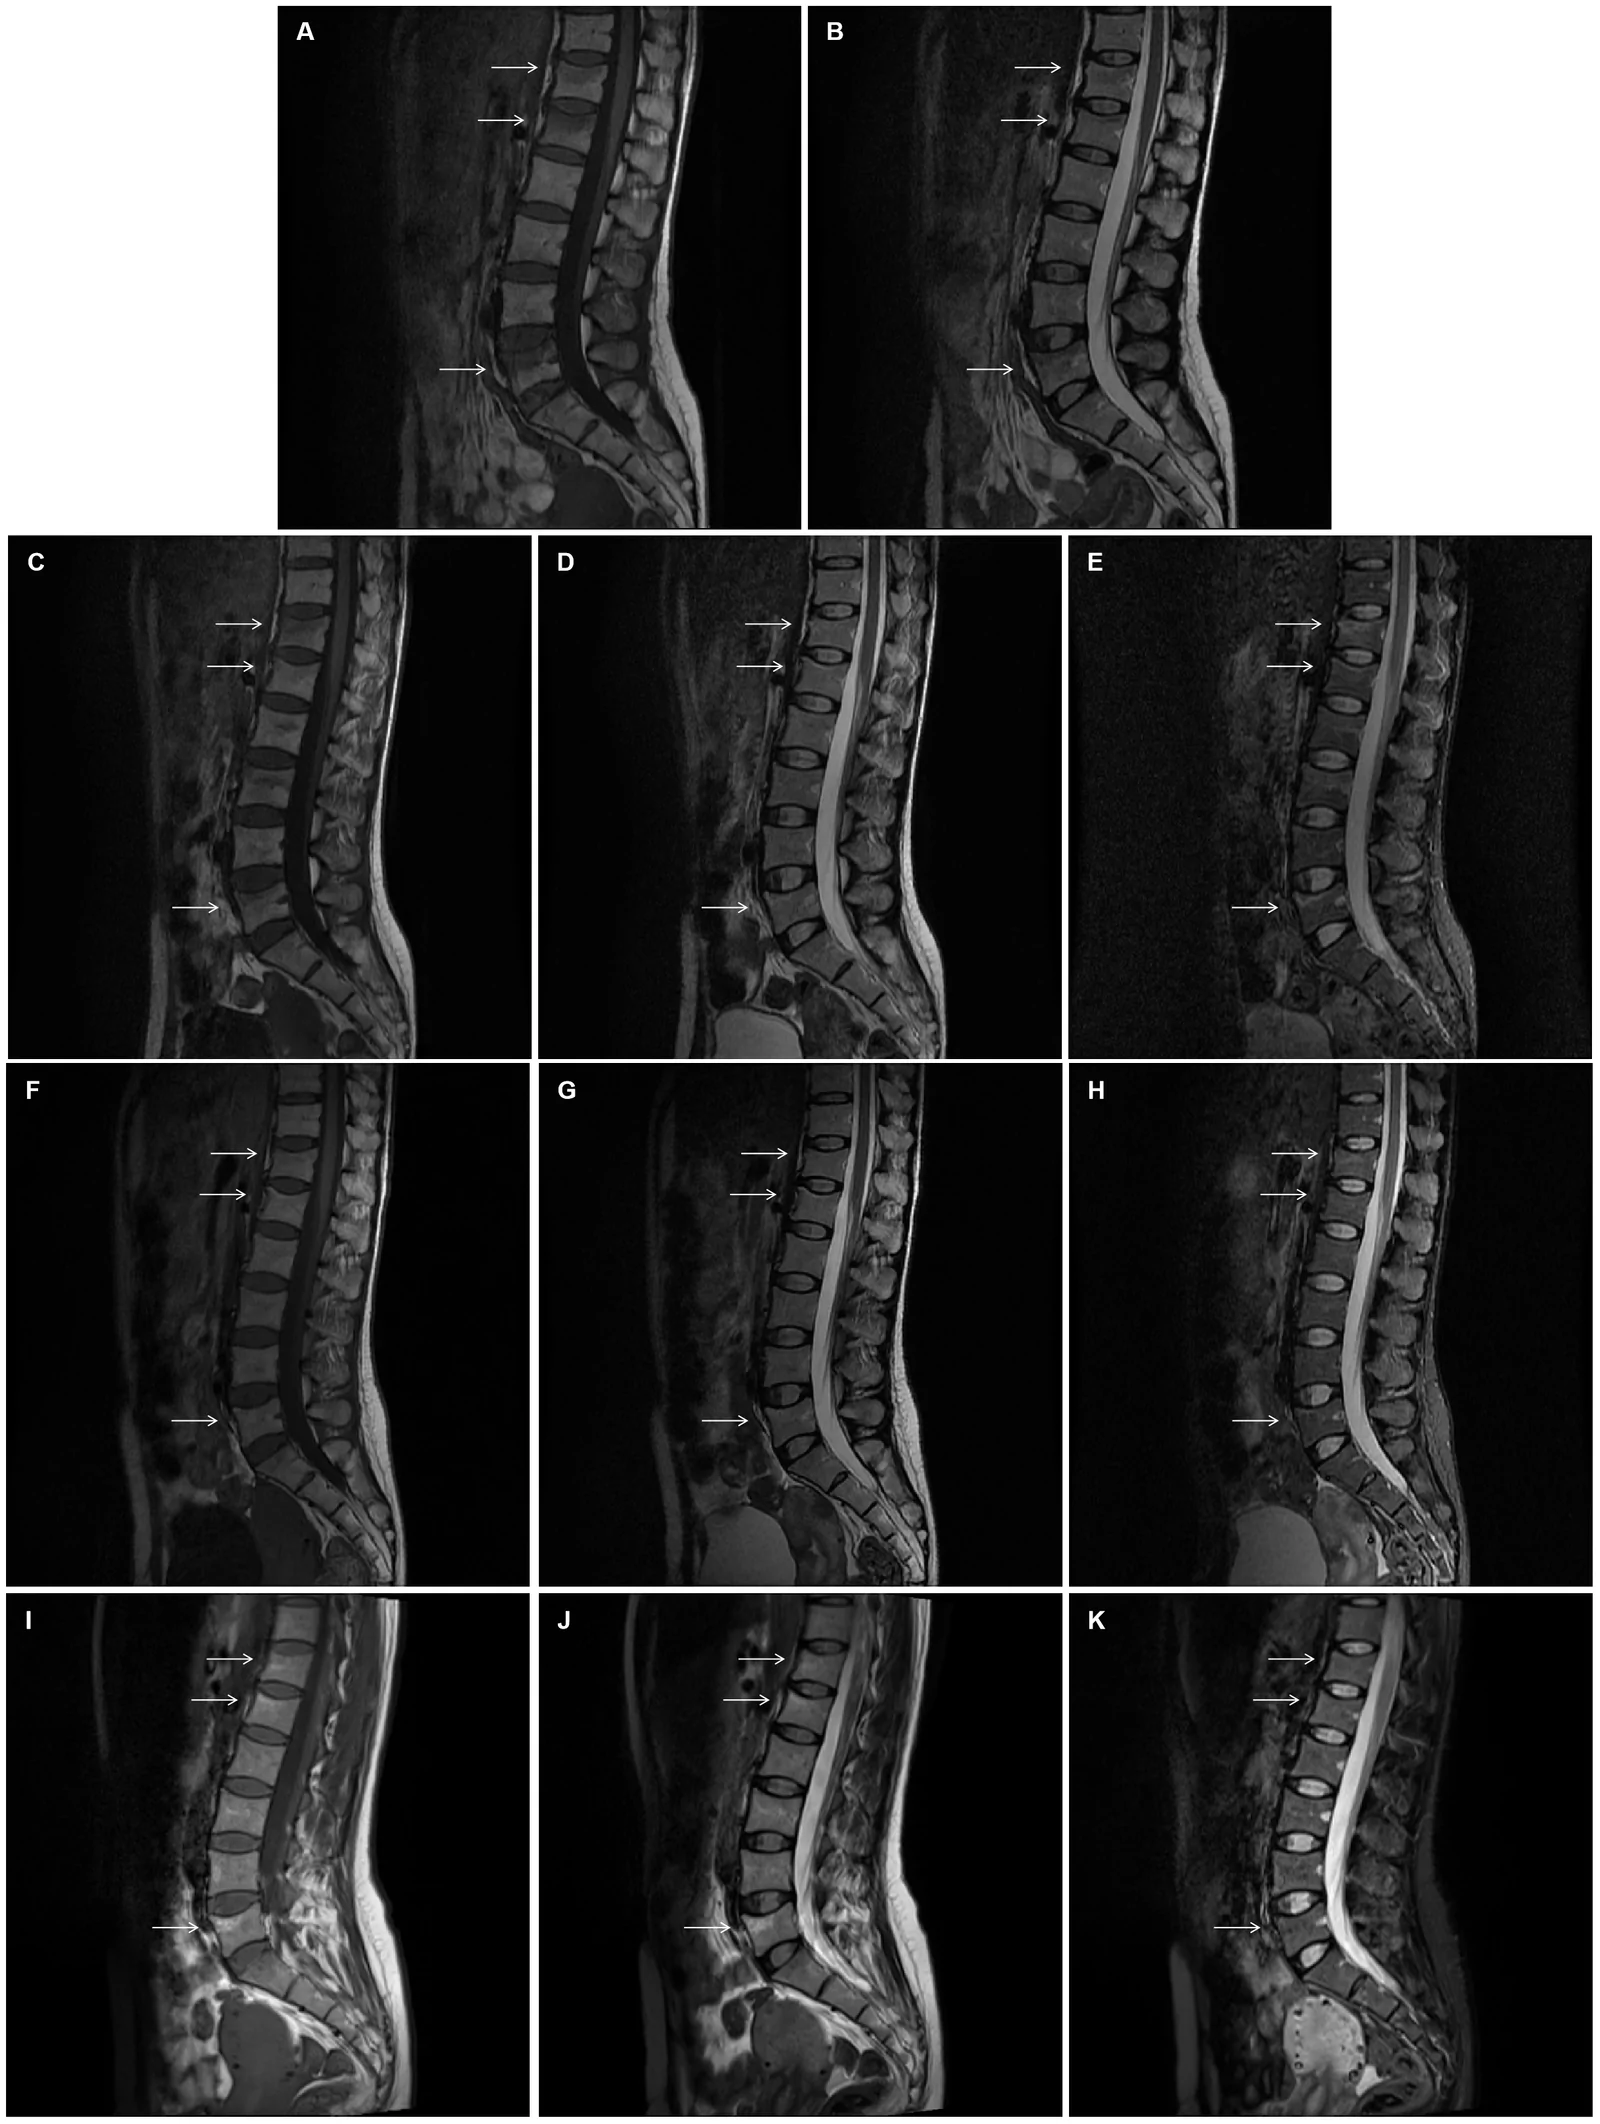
Early, post-treatment, and follow-up MRI of the thoracolumbar spine. (A) Sagittal T1-weighted MRI from June 6, 2021. (B) Sagittal T2-weighted MRI from June 6, 2021. (C) Sagittal T1-weighted MRI from July 2, 2021. (D) Sagittal T2-weighted MRI from July 2, 2021. (E) Sagittal STIR sequence from July 2, 2021. (F) Sagittal T1-weighted MRI from July 21, 2021. (G) Sagittal T2-weighted MRI from July 21, 2021. (H) Sagittal STIR sequence from July 21, 2021. (I) Sagittal T1-weighted MRI from March 3, 2022. (J) Sagittal T2-weighted MRI from March 3, 2022. (K) Sagittal STIR sequence from March 3, 2022.
